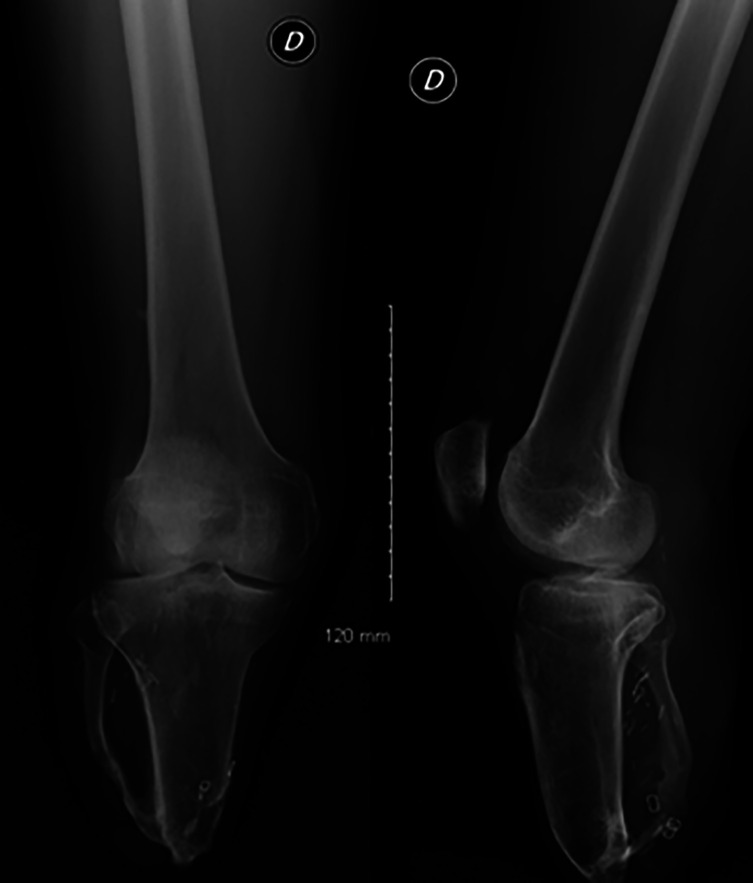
X-ray of the right knee showing the amputation level with a stump length of 12 cm.
